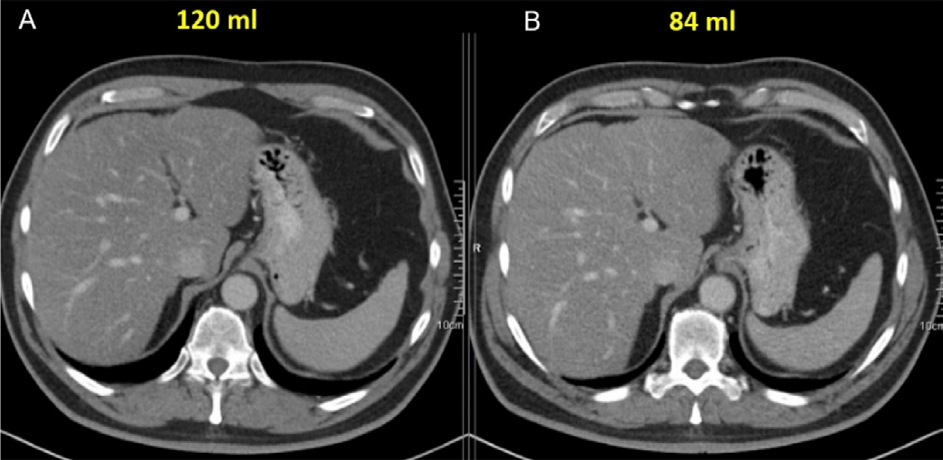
Portal venous phase contrast enhanced CT scans of the same patient. Oncology follow‑up patient. Scanned on two different scan dates (A & B) with an interval of six weeks. BMI = 31. A. Without contrast volume optimization (120 ml, VGA score 4). B. With contrast volume optimization (84 ml, VGA score 4).
